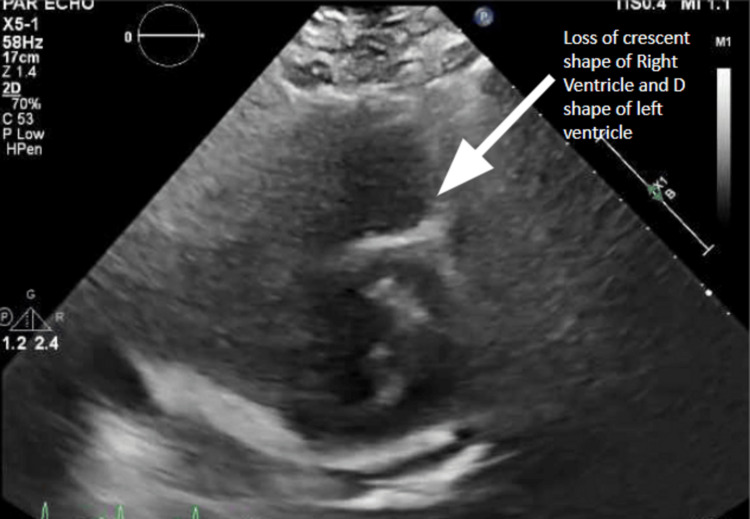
Transthoracic Echocardiogram Showing Parasternal Short Axis View With Loss of Normal Crescent Shape of Right Ventricle and D "Sign" (Cross Section of Left Ventricle Shaped Like the Letter "D")
